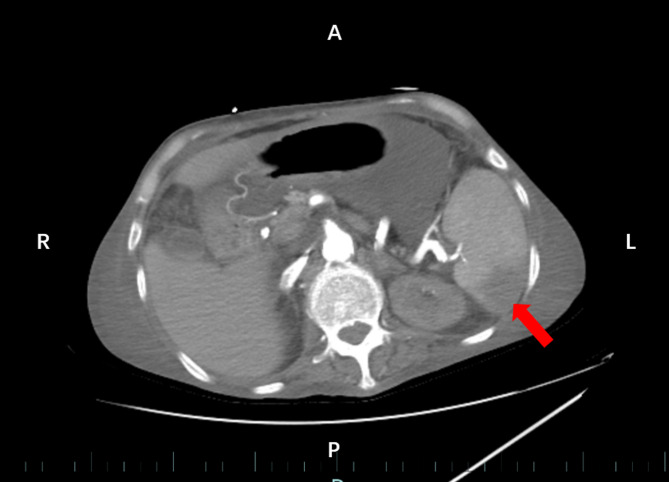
Abdominal CTA demonstrating splenic infarction and intestinal ischemic changes; the arrow indicates the infarcted area of the spleen
